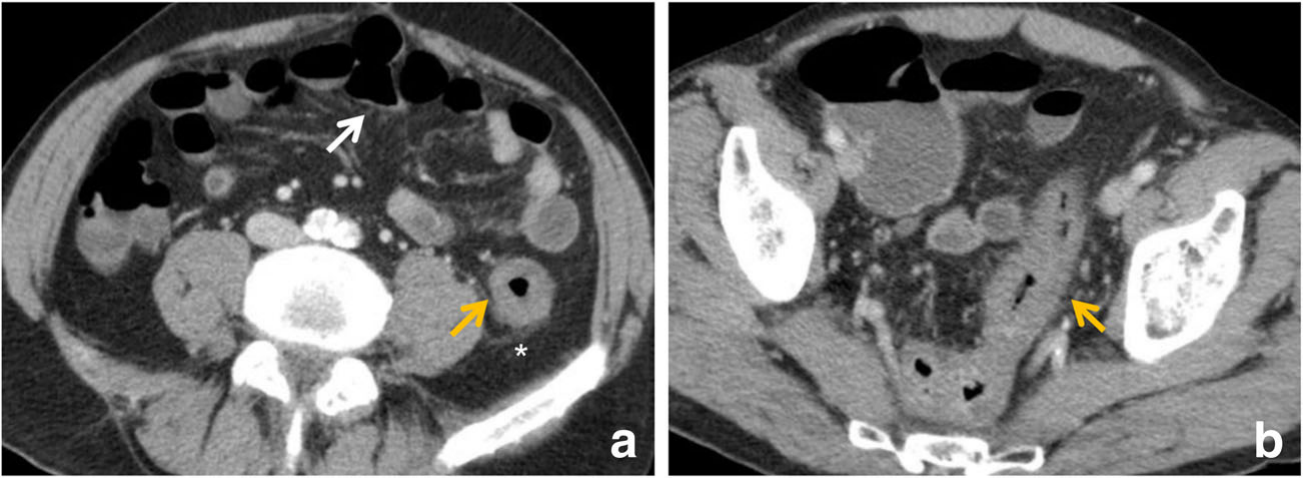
Ischaemic colitis in a 74-year-old male with vasculitis who presented with abdominal pain and bloody diarrhoea. (a, b) Contrast-enhanced CT scan reveals involvement of the sigmoid colon and splenic flexure (orange arrows) with marked wall thickening and pericolic streakiness (asterisk). Diagnosis was confirmed at colonoscopy and biopsy. The ischaemic process resolved
